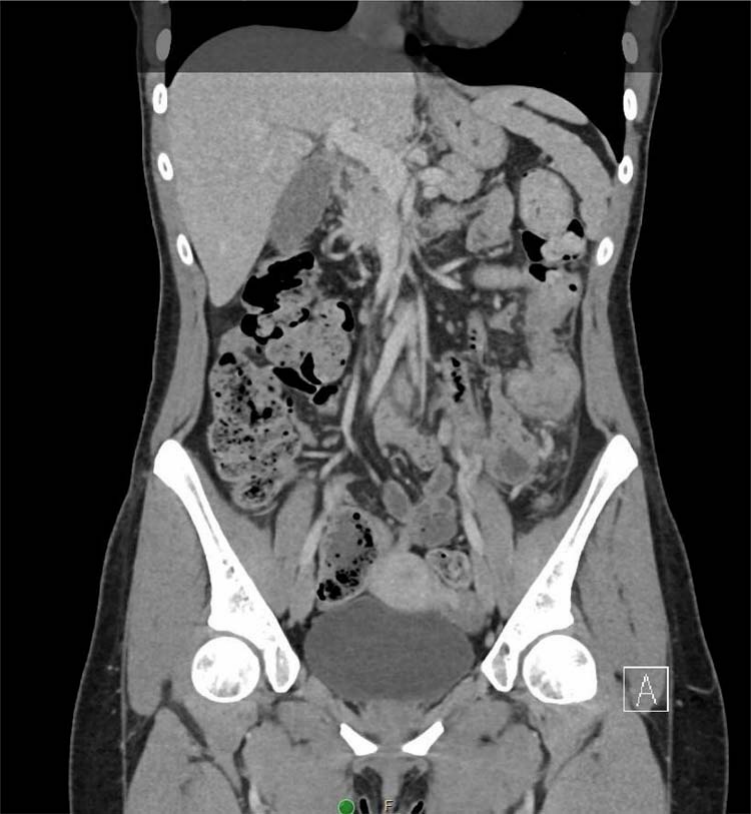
Coronal CT demonstrating segmental wall thickening of the descending colon with adjacent inflammation favoring acute diverticulitis or segmental colitis.
